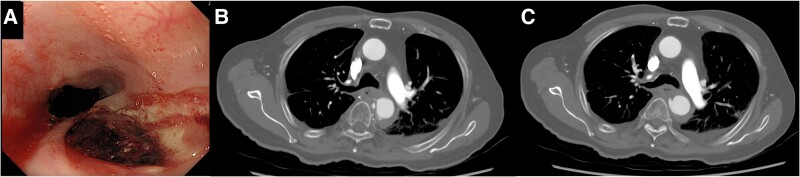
Gastrofiberscopy revealed an ulcer in the thoracic oesophagus (*A*), and computed tomography revealed that the same area was adjacent to the thoracic aorta (*B* and *C*).
